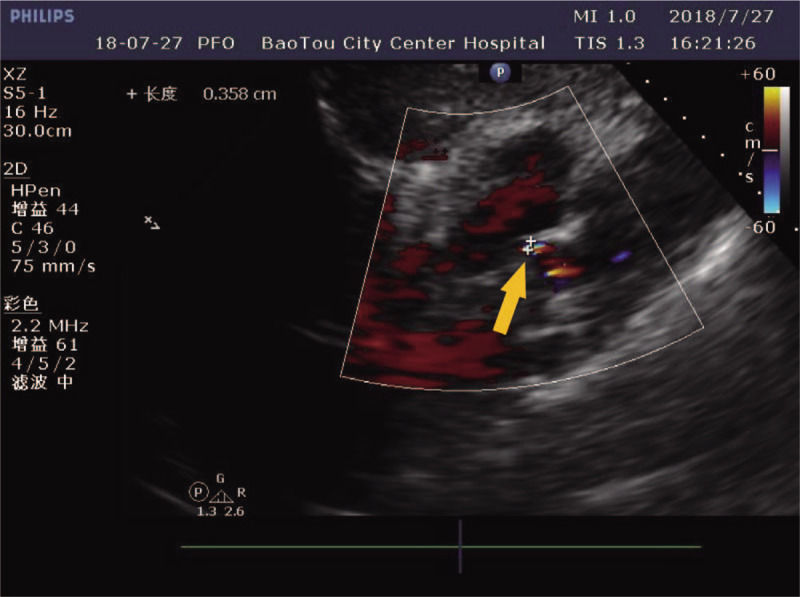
Transthoracic echocardiography showing shunt from PFO (yellow arrow). Images obtained 6 days after the femur fracture.
